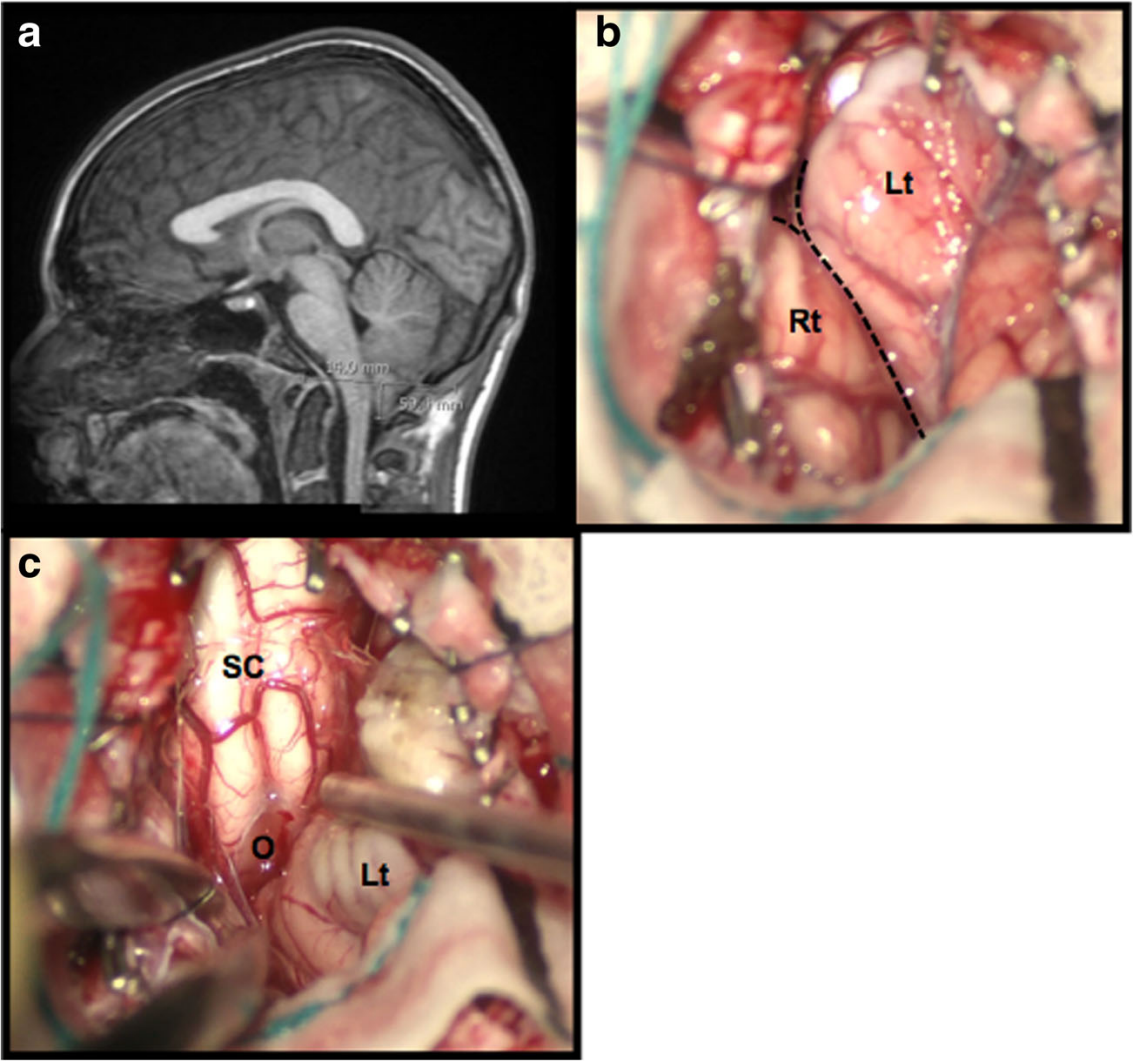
a Sagittal MRI showing caudal descent of the cerebellar tonsils. b–c Intra-operative images following suboccipital craniotomy and removal of the posterior arch of C1. Large cerebellar tonsils can be seen (separated by dashed line). The left tonsil - Lt is causing lateral displacement of the right tonsil (Rt) (b). Following coagulation, the tonsils have been reduced in size allowing exposure of the dorsal spinal cord (SC) and the obex of the 4th ventricle (O) (c)
